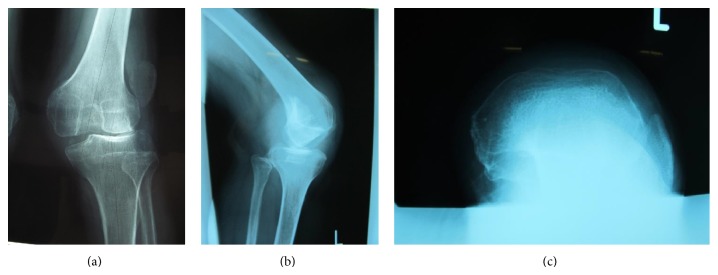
Preoperative radiographs: anteroposterior view (a), lateral view (b), and skyline view (c) of left knee.
